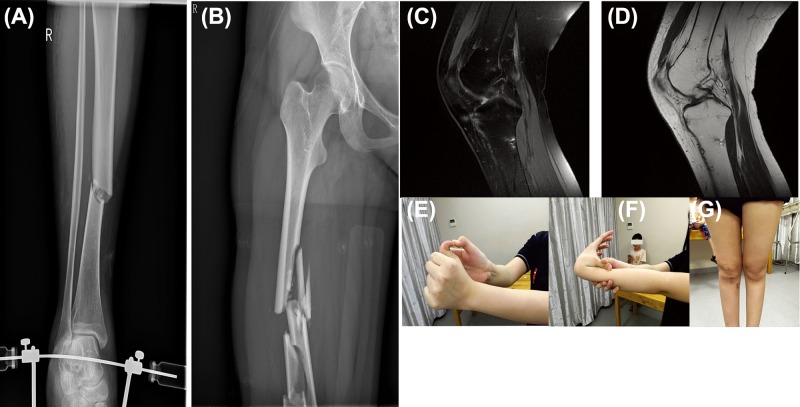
Radiographic images, magnetic resonance imaging, and photographs of the findings in proband III-1 Radiographic images: (A) right tibia fracture, (B) right femoral fracture. (C,D) Magnetic resonance imaging (MRI) of the posterior cruciate ligament rupture in the right knee-joint. Photographs of joint hypermobility (E,F), atrophic scarring (G).
